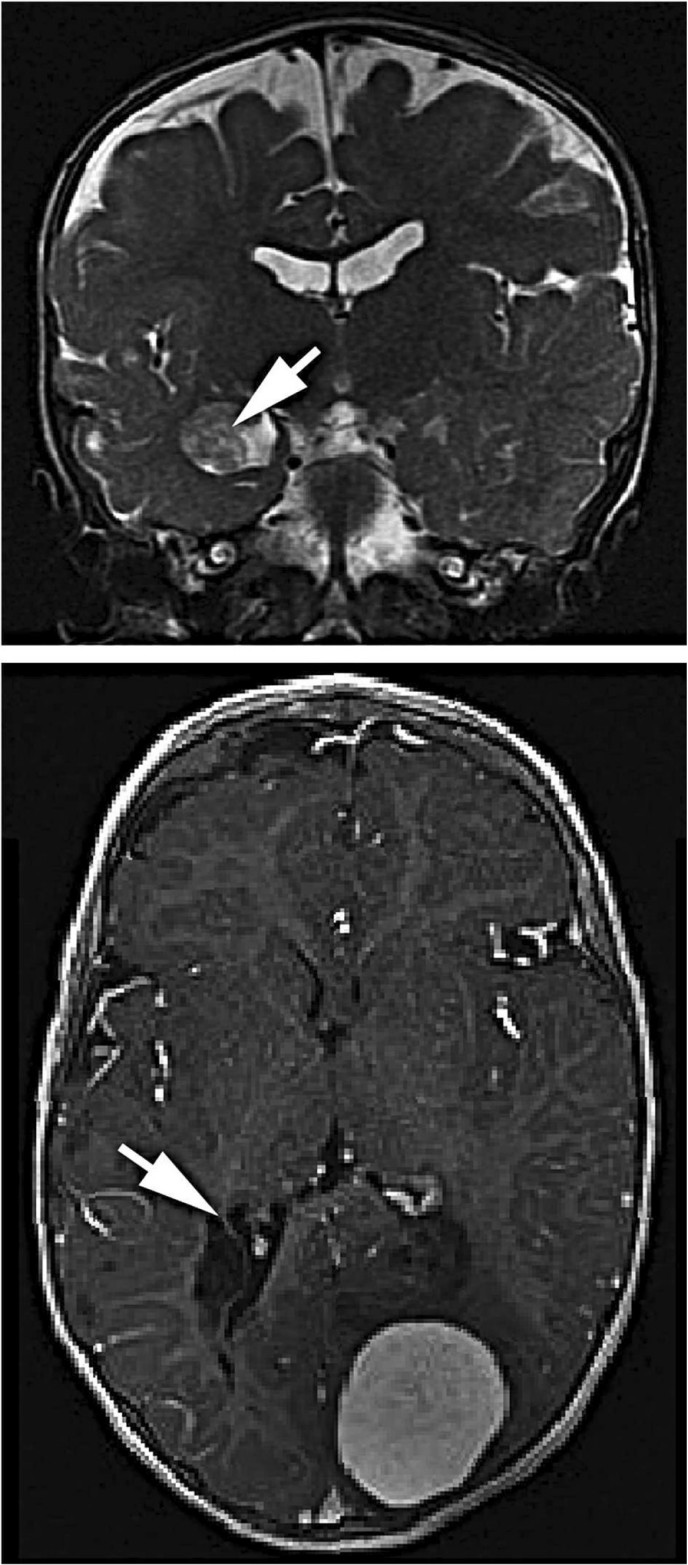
Magnetic resonance image of the brain. At 14 months age (upper image, coronal, T2 weighted) an enlarged left temporal lobe and a tumor of the right choroid plexus (arrow) were apparent. At 23 months age (lower image, horizontal, T1-weighted) a 4 cm tumor is present in the left occipital lobe. Periventricular cysts are located adjacent to the right occipital horn (arrow).
